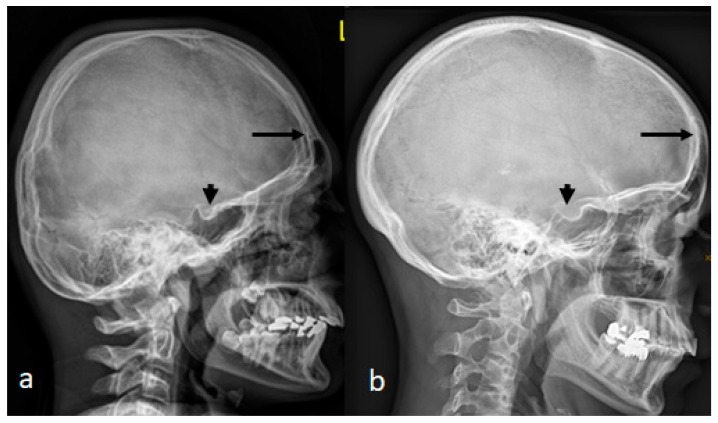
(a) AP skull radiograph of a-12-year-old boy showed brachycephalic skull secondary to craniosynostosis occurred because of bilateral early ossification of the coronal sutures, leading to bi-coronal craniosynostosis. Note the ossified interclinoid ligament of the sella turcica. (b) A lateral skull radiograph of a 38-year-old mother with a history of poor schooling achievements showed a very similar cranial contour of brachycephaly and massive ossification of the clinoid ligament of the sella turcica.
